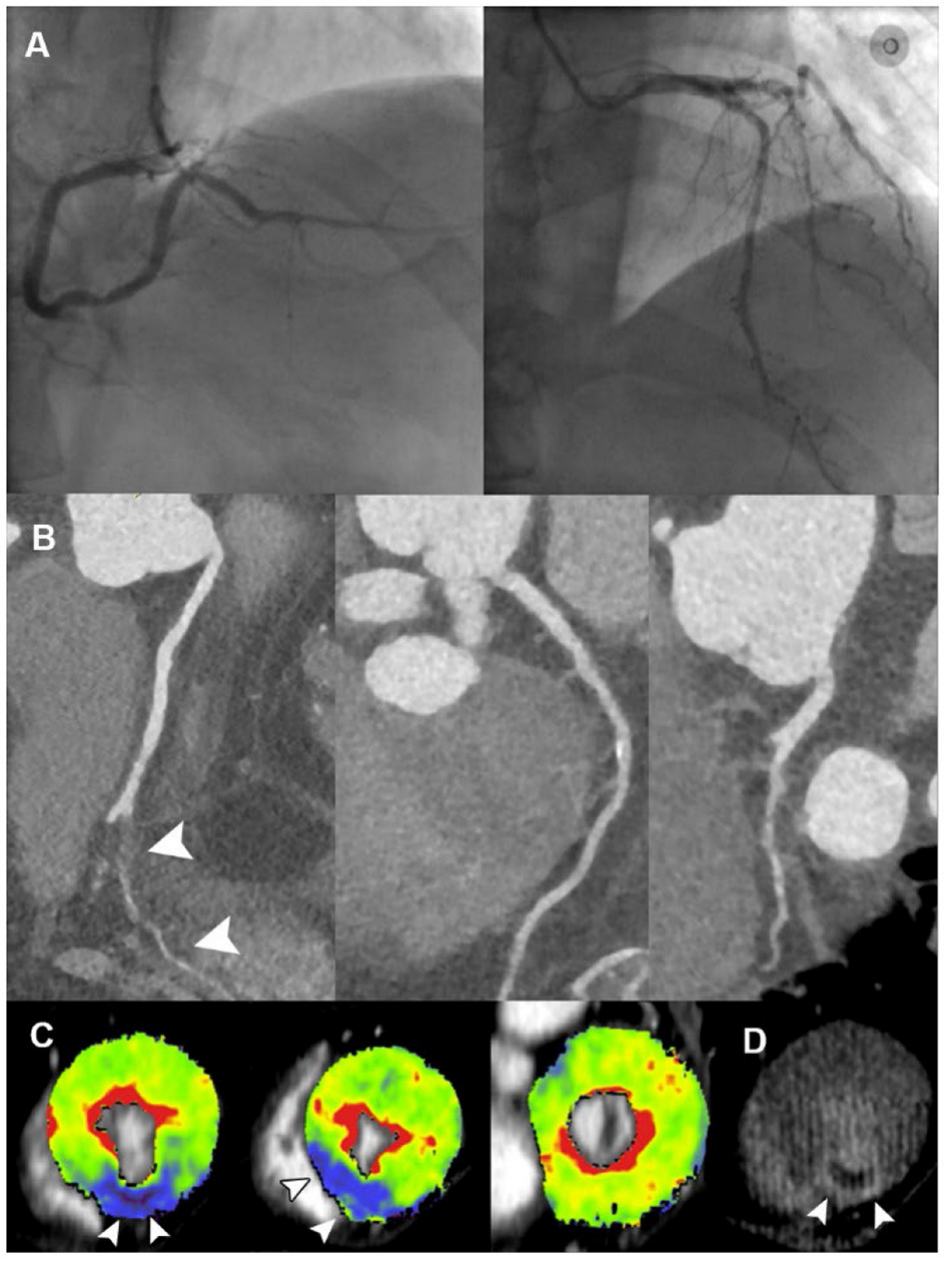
Representative case of vessel occlusion with MVO in a 66-year-old male with ACS and non-obstructive ICA. (A) ICA revealed mild stenosis at middle LAD and distal RCA, without presence of obstructive lesion. (B) CPR images showed occlusion of PDA ostium (white arrowhead). (C) Short-axis views of CT-MPI showed significantly reduced myocardial blood flow within PDA territory (white arrowhead). (D) LIE image showed the transmural late enhancement with the presence of MVO within PDA territory (white arrowhead). ACS, acute coronary syndrome; CPR, curved planar reformation; CT, computed tomography; ICA, invasive coronary angiography; LAD, left anterior descending; LIE, late iodine enhancement; MPI, myocardial perfusion imaging; MVO, microvascular obstruction; PDA, posterior descending artery; RCA, right coronary artery.
